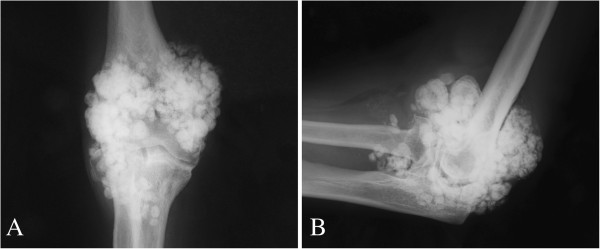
Anteroposterior (A) and lateral (Bx) radiographs of the left elbow show multiple rounded, calcified bodies widespread throughout the elbow joint.
